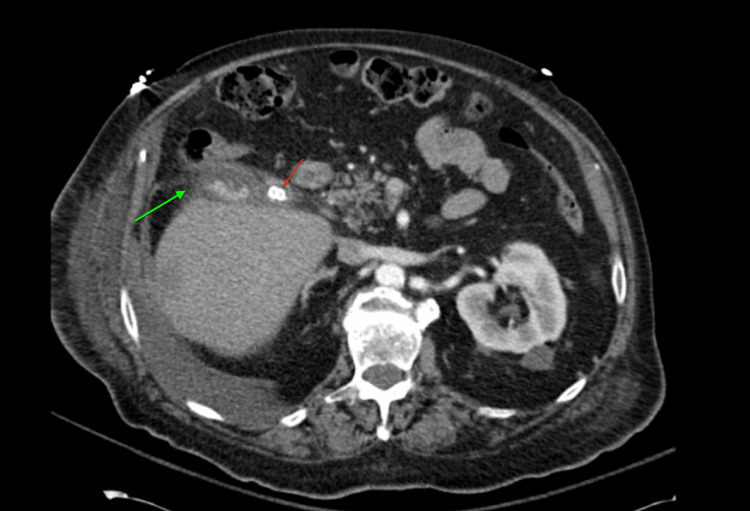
Axial CT with contrast showing pericholecystic fat stranding (green arrow) and cholelithiasis (red arrow).
